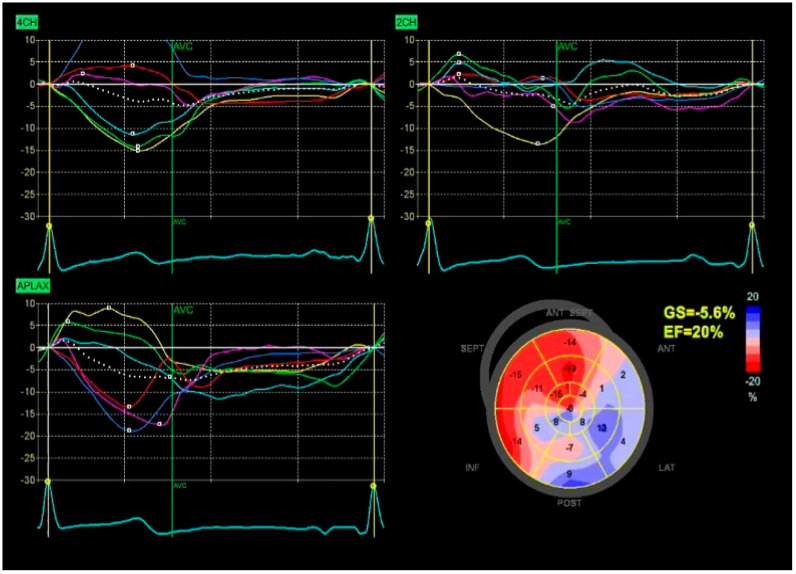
Global longitudinal strain (GLS) analysis in a patient with severe left ventricular dysfunction. Speckle-tracking echocardiography from apical views shows markedly reduced strain in most myocardial segments. The bull’s-eye plot highlights global impairment (GLS –5.6%, normal ≤ –18%), with predominant septal and inferior segment involvement. Calculated EF is 20%, consistent with advanced systolic failure.
